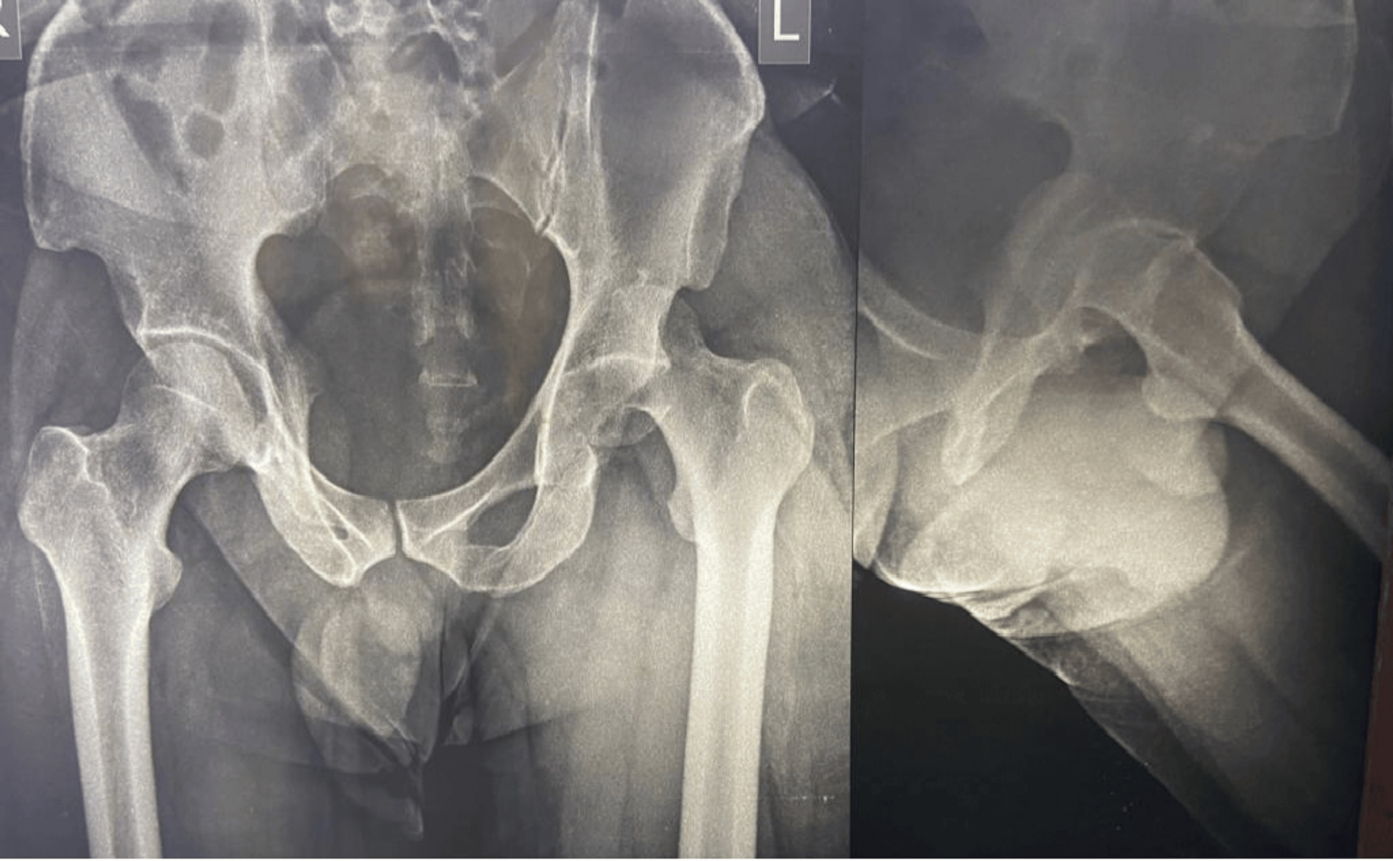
X-ray both hips anteroposterior (AP) and lateral (Lat) view of case no 2
